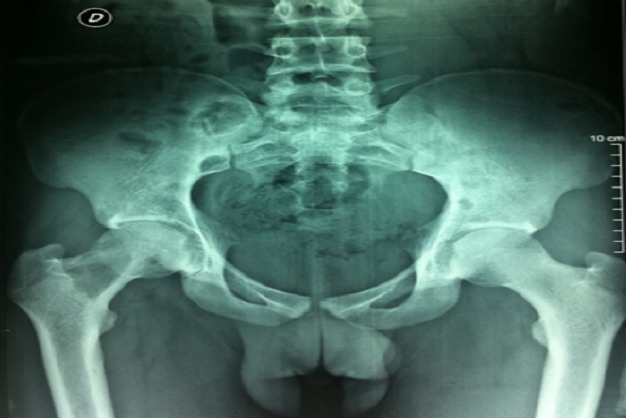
Radiographie du bassin a montré la présence d'une image lytique du col fémoral droit avec respect de la corticale
